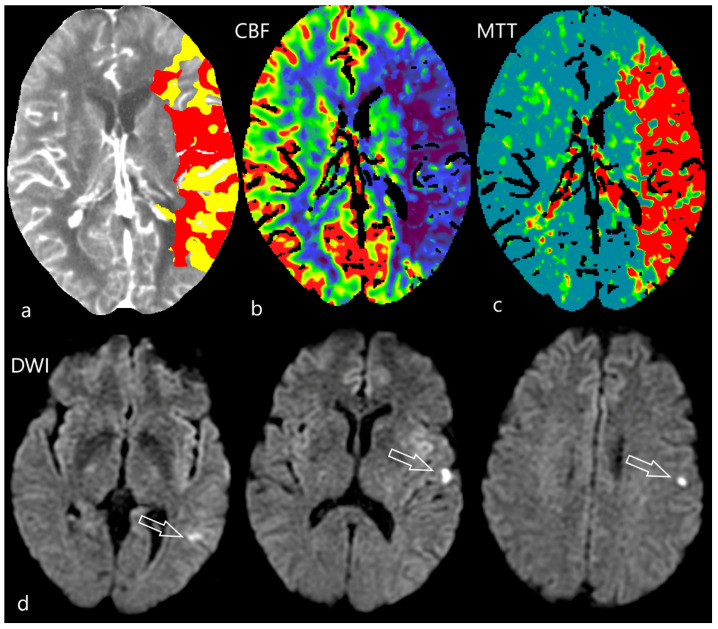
Ghost infarct on CTP. 68-year-old female was referred as acute stroke in the early time window 1.5 h since the last known well. She suffered from right sided hemiparesis and aphasia. CTA confirmed occlusion of the left medial cerebral artery. On CTP [upper row, (a)] there is a map with the calculation on penumbra (yellow area) and ischemic core (red area). On the cerebral blood flow map (CBF) a hypoperfusion of the whole region of the left medial cerebral artery was seen [ (b), blue area], mean transit time (MTT) was prolonged [(c), red area]. Endovascular treatment was promptly indicated. The second day after endovascular mechanical thrombectomy, she was without neurological deficit and was sent to MRI follow-up [lower row, (d)]. On diffusion weighted images (DWI) only 3 very small acute ischemic lesions were found (arrows).
